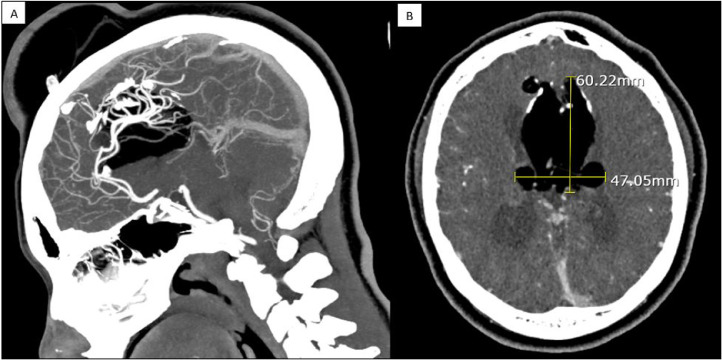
CT angiography of brain from June 2021. (A) Coronal view depicting blood vessel going through the lipoma. (B) Axial view show casing the measurement of the lipoma 6 cm by 4.7 cm.
